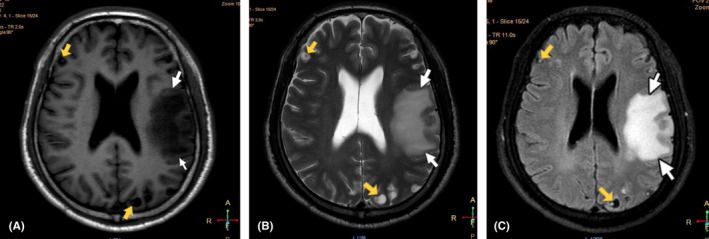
MRI brain. (A) Axial T1 weighted View. (B) Axial T2 weighted View. (C) Axial Flair View. White arrows showing marked amount of edema in left parietal and temporal lobes, Yellow arrows showing cystic lesions in bilateral cerebral and cerebellar hemispheres
